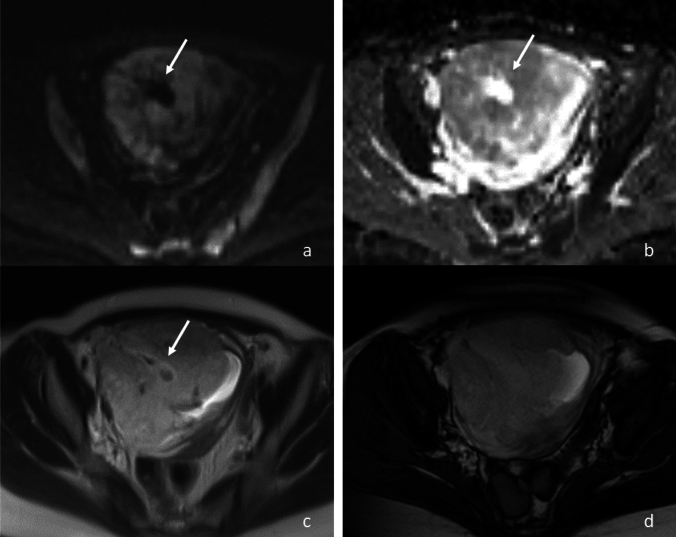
A 39-year-old woman with placenta previa and placenta accreta. a Axial diffusion-weighted image shows a hypointense area (arrow) in the placenta. b The apparent diffusion coefficient map shows hyperintense space (arrow) corresponding to the hypointense diffusion area in (a), indicating diffusion lacuna. c Axial T2-weighted half-Fourier acquisition single-shot turbo spin echo shows faint flow void in the diffusion lacuna (arrow). d True fast imaging in steady-state precession shows high signal intensity throughout the placenta, including the diffusion lacuna
